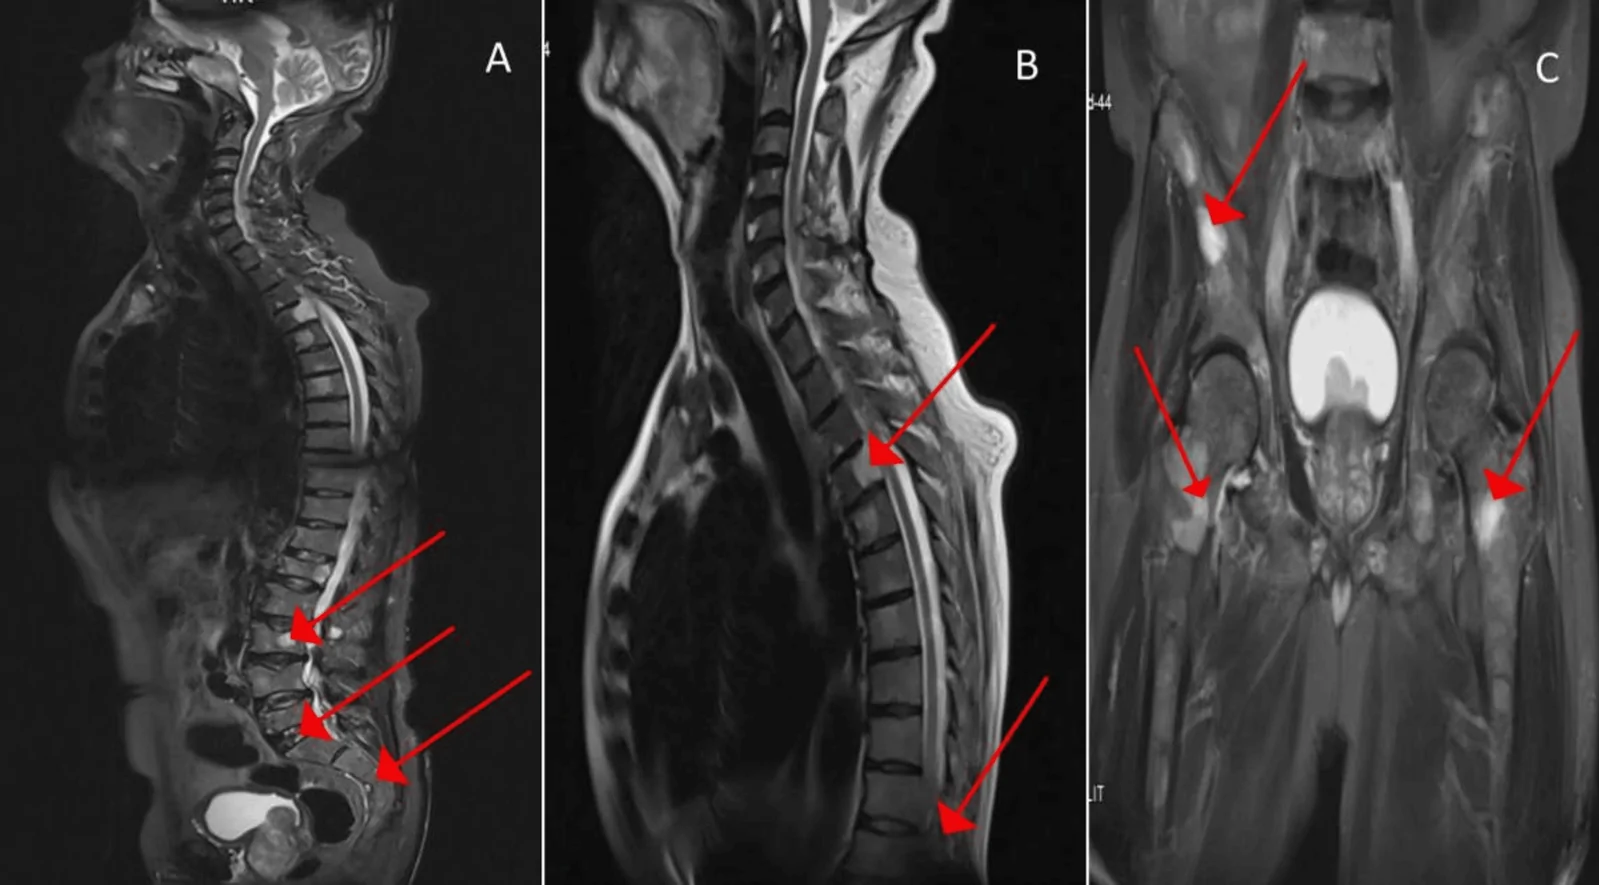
Whole-body MRI (coronal and sagittal acquisition of the spinal axis, pelvis, femurs, sternum, and shoulder girdles) Sequences weighted in T1, STIR, and DWI. Analysis according to MY-RADS criteria. Whole-body diffusion MRI shows bone marrow infiltration suggesting an active mixed-type myeloma, both focal and diffuse, according to the MY-RADS classification, with vertebral compression; an expansive process of the sphenoid bone requires follow-up orbital-brain MRI for better morphological assessment. (A-B) Nodular and patchy diffuse infiltration affecting the axial skeleton, with high T2 and STIR signal, and compression fractures of the vertebrae at D12, L1, L3, and L5 (arrows). (C) Nodular and patchy diffuse infiltration affecting the pelvis and femur, with high T2 and STIR signal (arrows). STIR: short tau inversion recovery; DWI: diffusion-weighted imaging; MRI: magnetic resonance imaging
